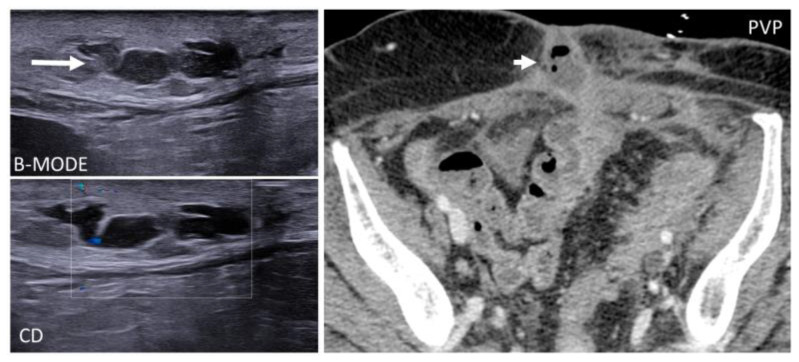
Abscesses on US and CT in a 69-year-old woman with myelofibrosis, presenting with pain along the surgical scar and persistent fever two weeks after splenectomy. B-mode US (first picture, arrow) demonstrates a fluid collection, with no significant vascularization on color Doppler (CD, lower picture). On portal-phase CT (third picture), the abscess is easily distinguishable (arrowhead).
